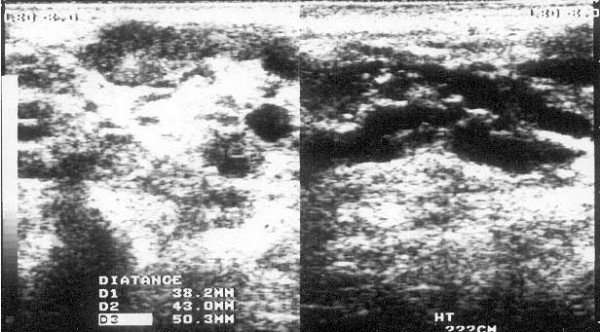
Ultrasound scan of axilla after therapy showing collapsed lymphatics (in longitudinal and transverse axis).
